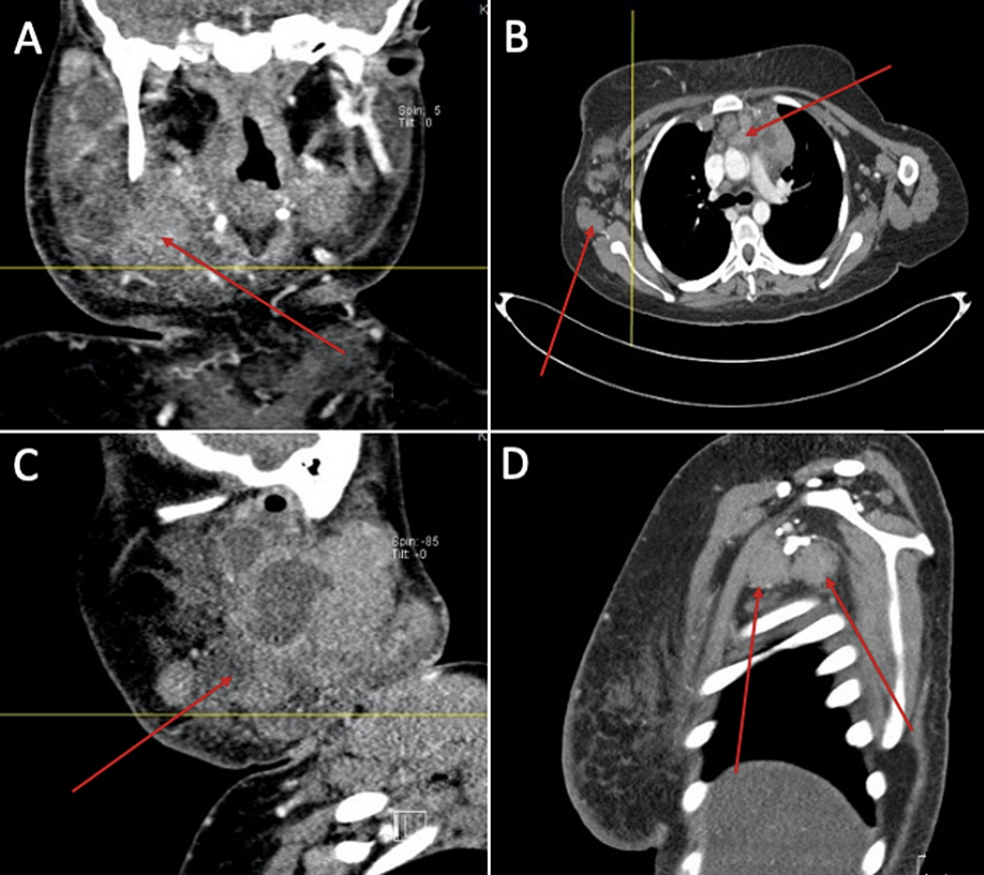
CT scan of an 18-year-old female showing multiple enlarged lymph nodes. A: Neck CT scan, coronal view, showing enlarged right cervical lymph node. B: Chest CT scan, axial view, showing multiple enlarged lymph nodes. C: Neck CT scan, sagittal view, showing enlarged right cervical lymph node. D: Chest CT scan, sagittal view, showing enlarged lymph nodes.
